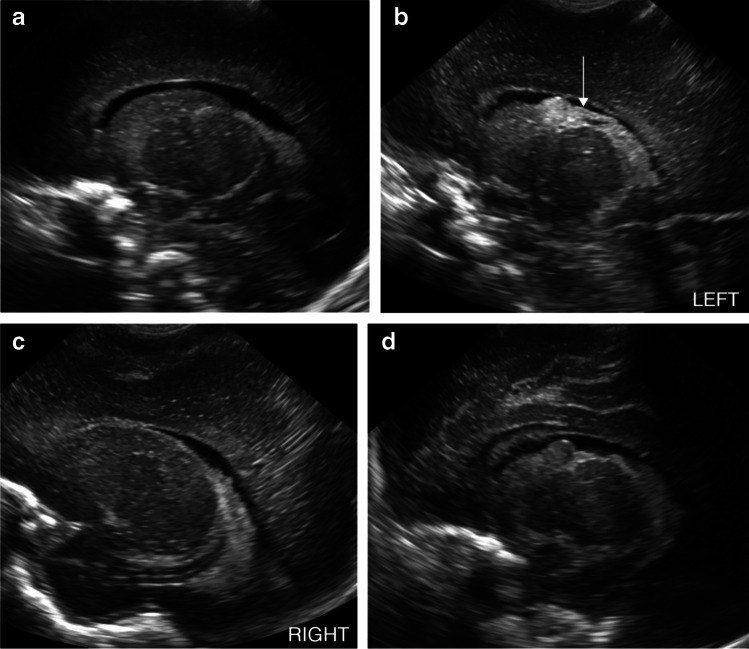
A sagittal image from a normal head ultrasound (US) at 7 days of life in a boy born at 26 weeks’ gestational age (a). A sagittal image from a follow-up US at 29 days shows new grade 2 intraventricular hemorrhage on the patient’s left (*arrow*) (b). A comparison sagittal image of the patient’s normal right side on a follow-up US at 29 days (c). A sagittal image from a subsequent US at 36 days shows decreased echogenicity of the grade 2 intraventricular hemorrhage on the patient’s left compatible with expected evolution of hemorrhage (d)
